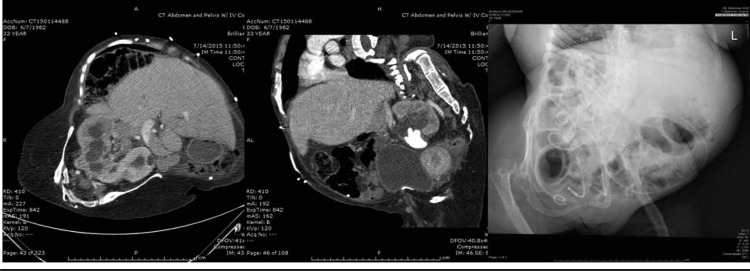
Selected radiographic images displaying complex anatomy. From *left* to *right*: axial CT image with pelvic horseshoe kidney; sagittal CT image showing spinal curvature; radiograph showing skeletal deformities.
